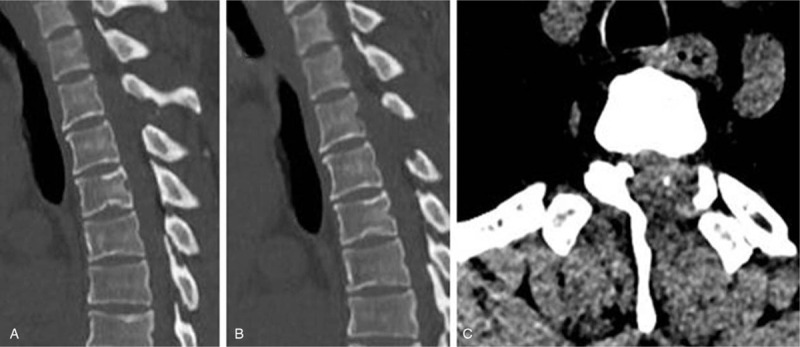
CT on the first day after surgery: sufficient resection of the T2 and 3 ossification of the ligamentum flavum.
